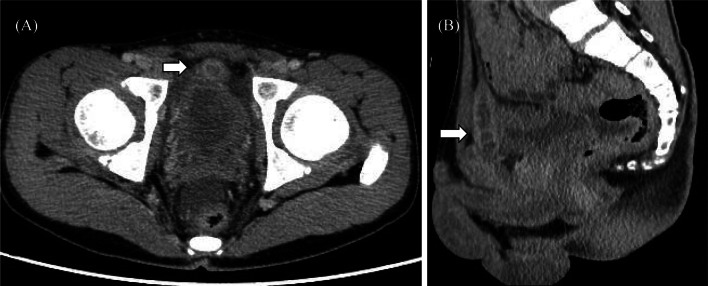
Computed tomography revealed an abscess inside a fistula lumen on the posterior surface of the rectus abdominis muscles in the midline of the lower abdomen (arrows) (A: axial B: sagittal)
